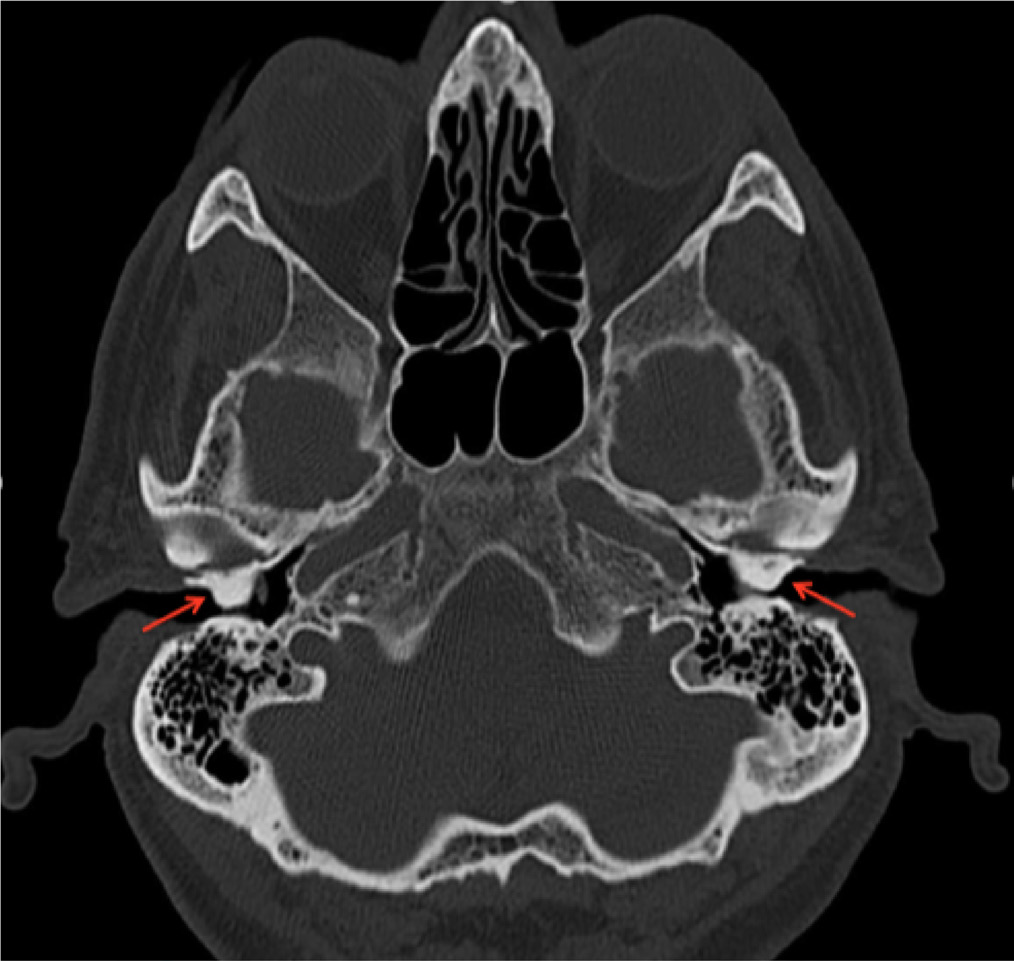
Axial high-resolution CT of the temporal bone demonstrating bilateral broad-based focal circumferential bony overgrowths (arrow) of the right and left osseous external auditory canal. These are causing significant narrowing of the lumen, with a residual luminal diameter of 1–2 mm bilaterally.
